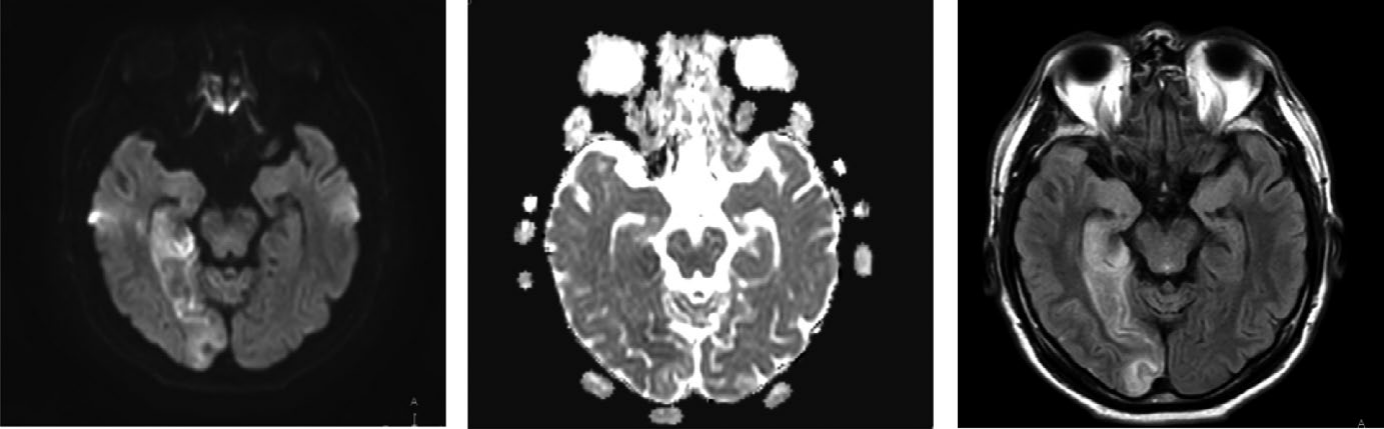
MRI images of the brain in axial view 10 days after stroke
